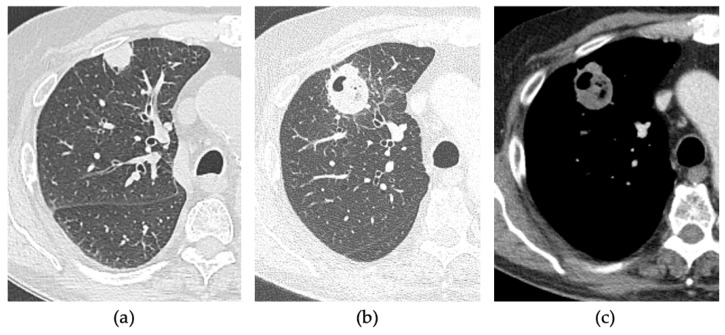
83-years-old man with NSCLC in the right upper lobe. (a) Axial pre-treatment CT. Axial 1-month follow-up CECT after MWA with VPS shows (b) a nodular-shaped margin of the consolidation of the ablation zone and (c) with the uptake of contrast, suggestive of residual disease.
